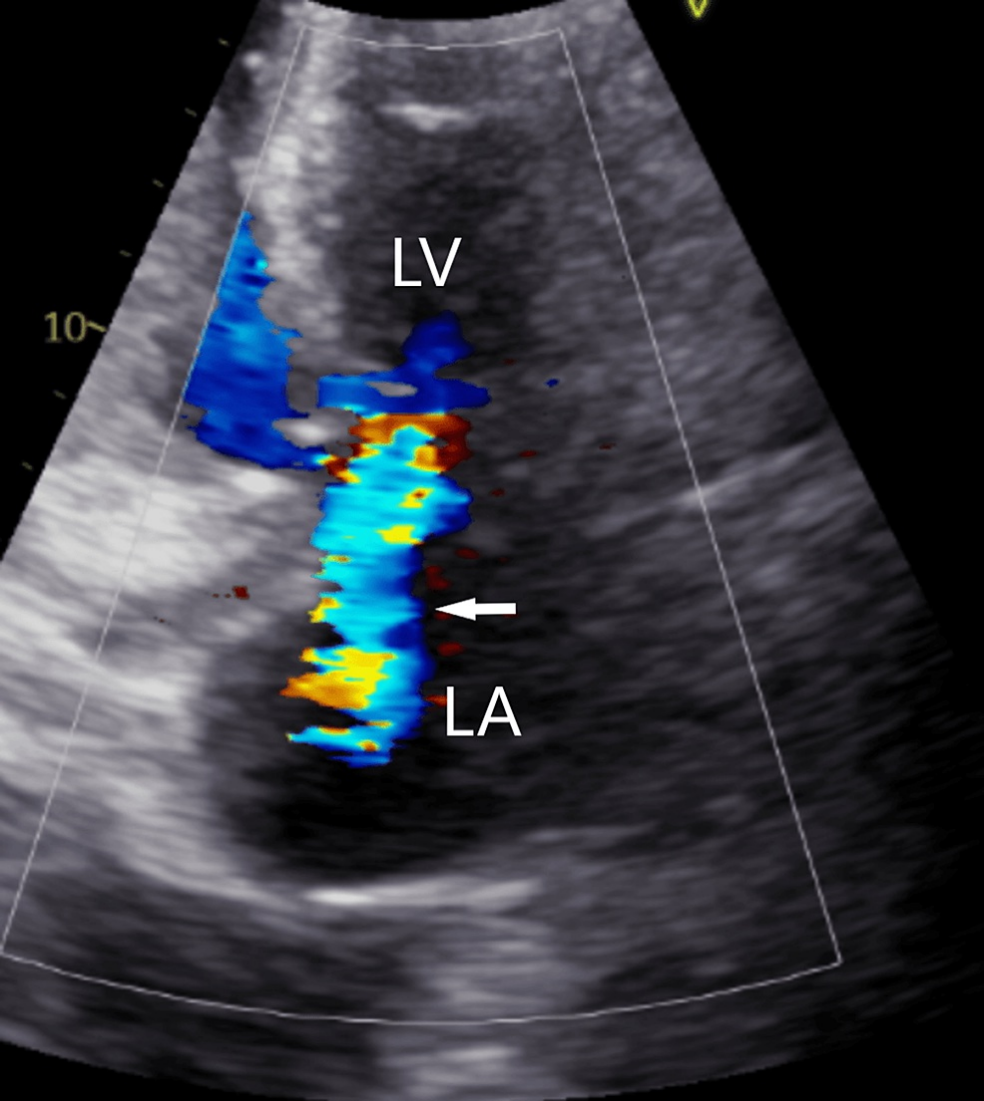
TTE showing moderate mitral regurgitation (arrow). LA, left atrium; LV, left ventricle; TTE, transthoracic echocardiogram
